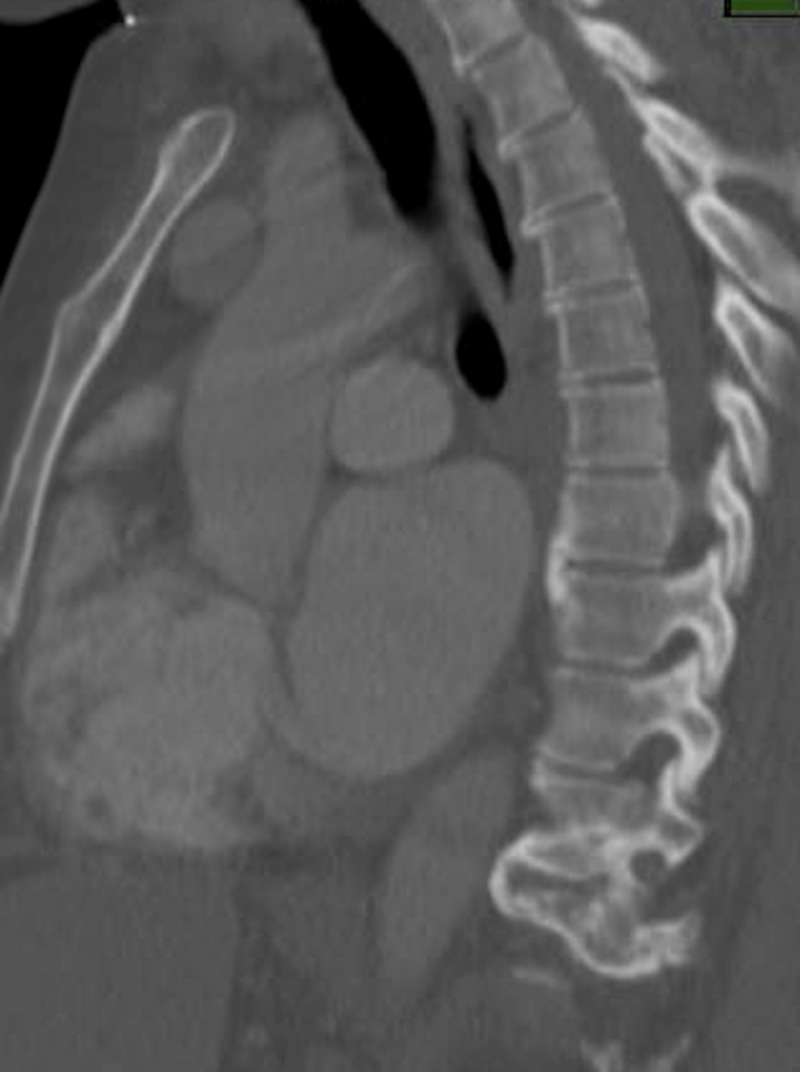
18-month preoperative CT scan depicting no evidence of disease CT (Computed Tomography)
